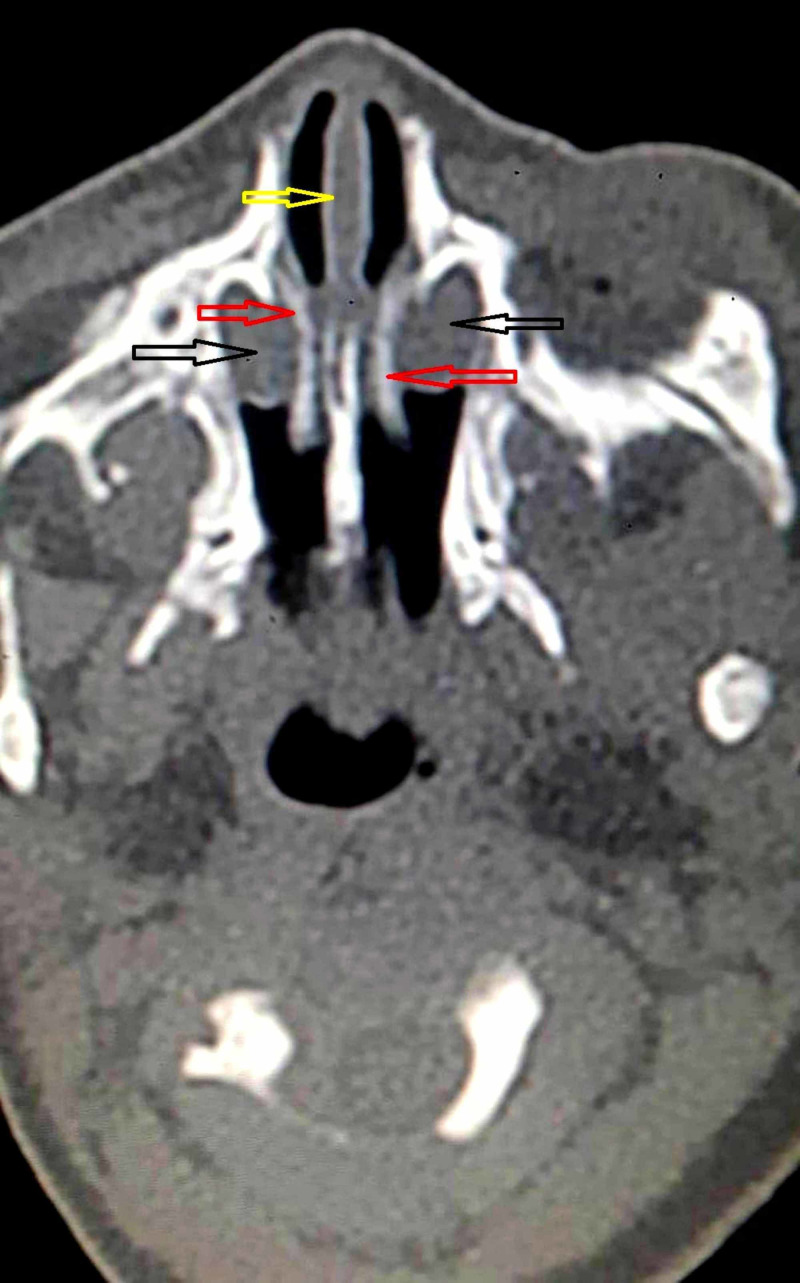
Non-contrast computed tomogram axial section showing intranasal cystic masses (black arrows) pushing inferior turbinates (red arrows) medially touching the nasal septum (yellow arrow) and completely obstructing bilateral nasal cavity.
